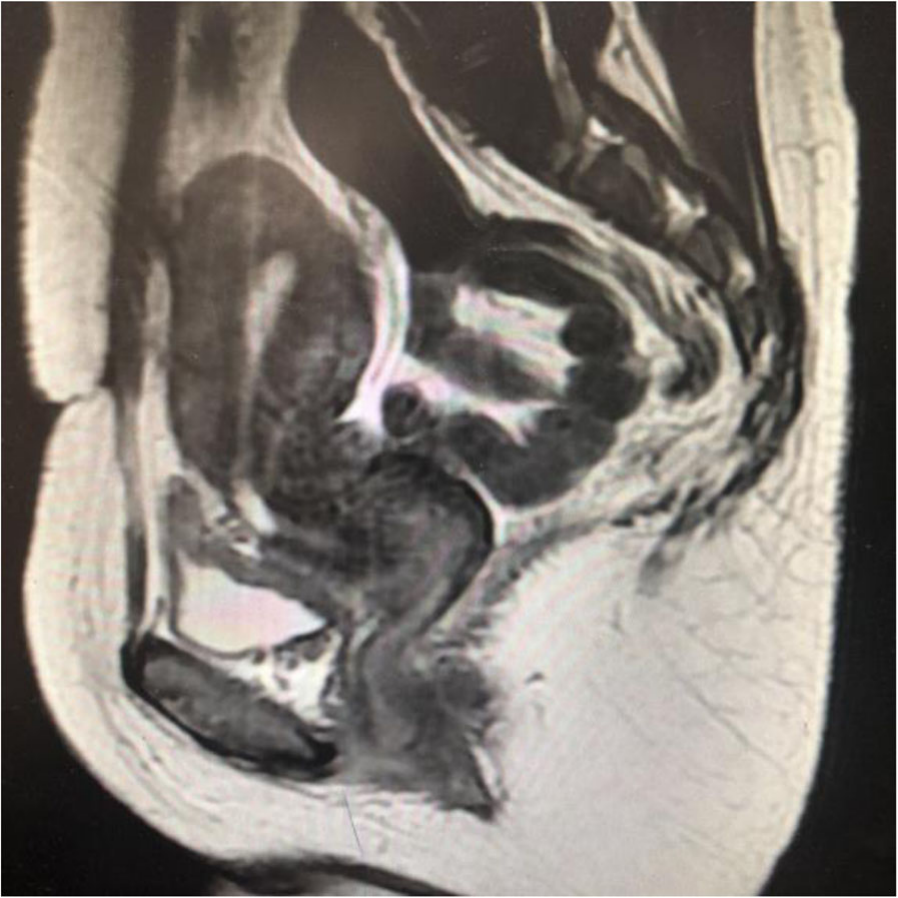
Preoperative picture via MRI
